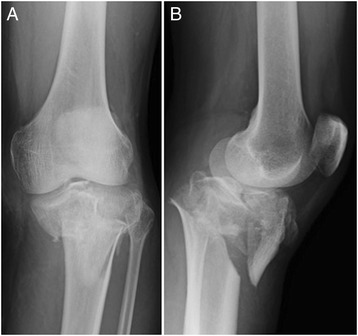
Anteroposterior (a) and lateral (b) radiography of the *left knee*. It was classified as an AO 41-C2 comminuted fracture of the tibial plateau
